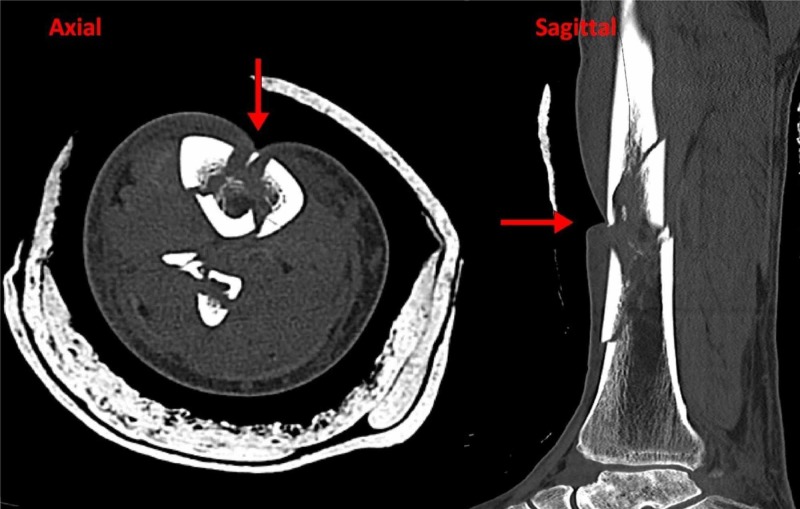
Axial and sagittal computed tomography (CT) images demonstrating tethering of the soft tissues within the fracture site prior to reduction
